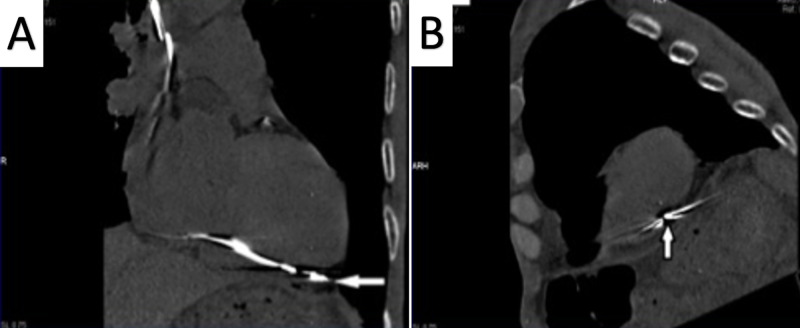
Non-contrast computed tomography of chest (oblique sagittal views) showing the lead tip (arrow) outside the right ventricular cavity and lying epicardially at the left ventricular apex in the pericardium
